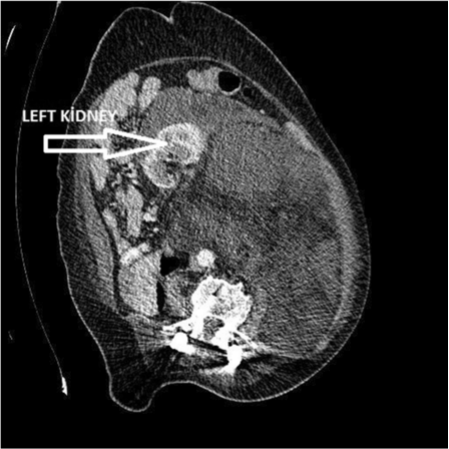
CT image of left kidney being pushed laterally and anteriorly to the right.
